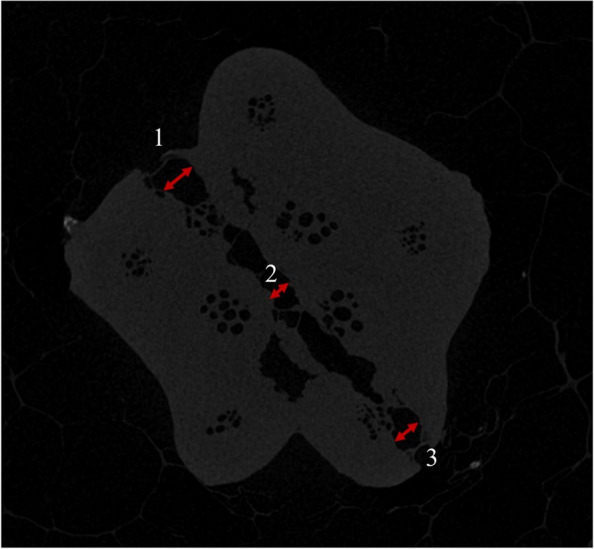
Micro CT and stem wound measurement. In the figure, 1, 2, and 3 are the initial, middle, and end positions of the puncture, respectively. The wound length is the average of the wound lengths at these three locations, mm
